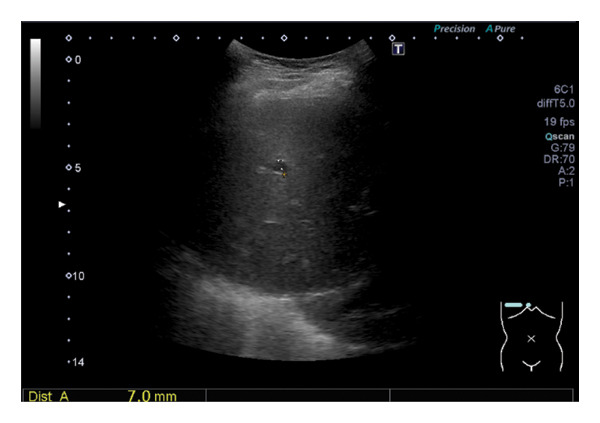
Abdominal ultrasonography findings during hospitalization. Presence of a 7 mm liver cyst in Segment VIII.
